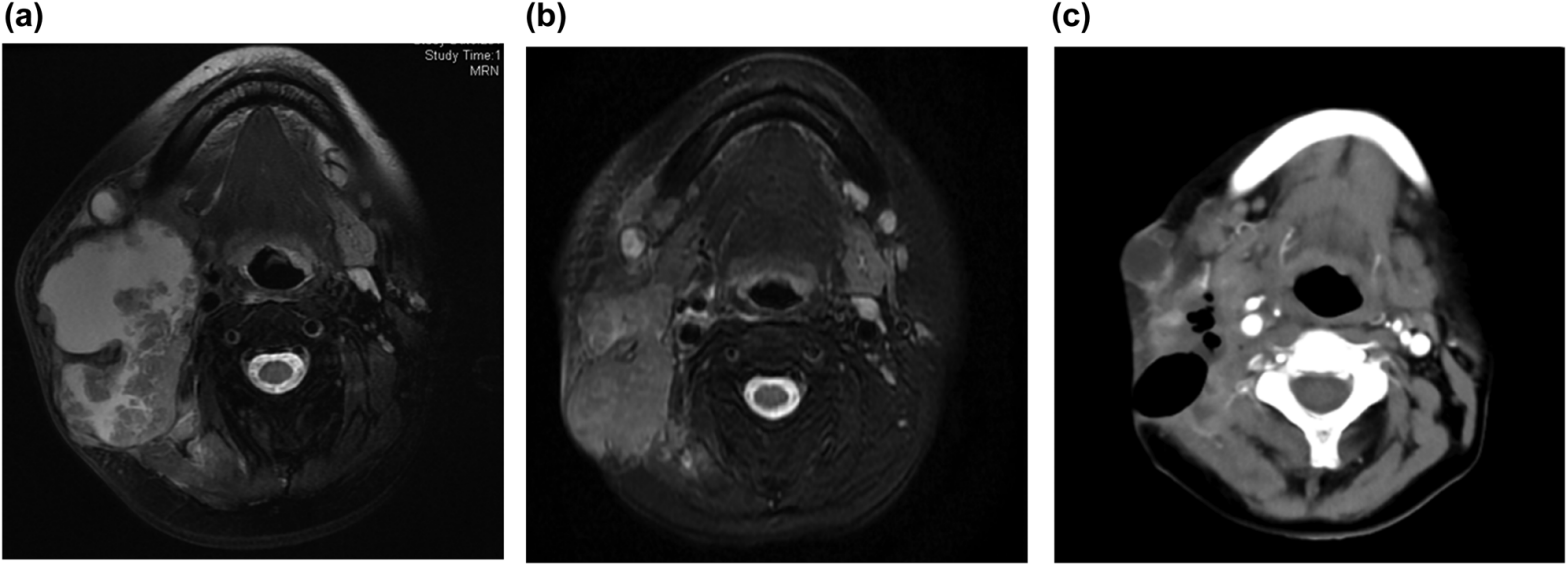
Images of neck tumor. (a) Before second-line chemotherapy, MRI revealed a soft tissue mass with a volume of about 7.5 cm^3^ × 5.5 cm^3^ × 7 cm^3^ on the right neck that invaded adjacent muscles and skin, and multiple enlarged cervical lymph nodes. (b) After the second cycle of second-line chemotherapy, MRI examination revealed a slight decrease in the volume of the tumor. (c) After apatinib treatment for 10 days, the computed tomography revealed the neck tumor was markedly reduced.
